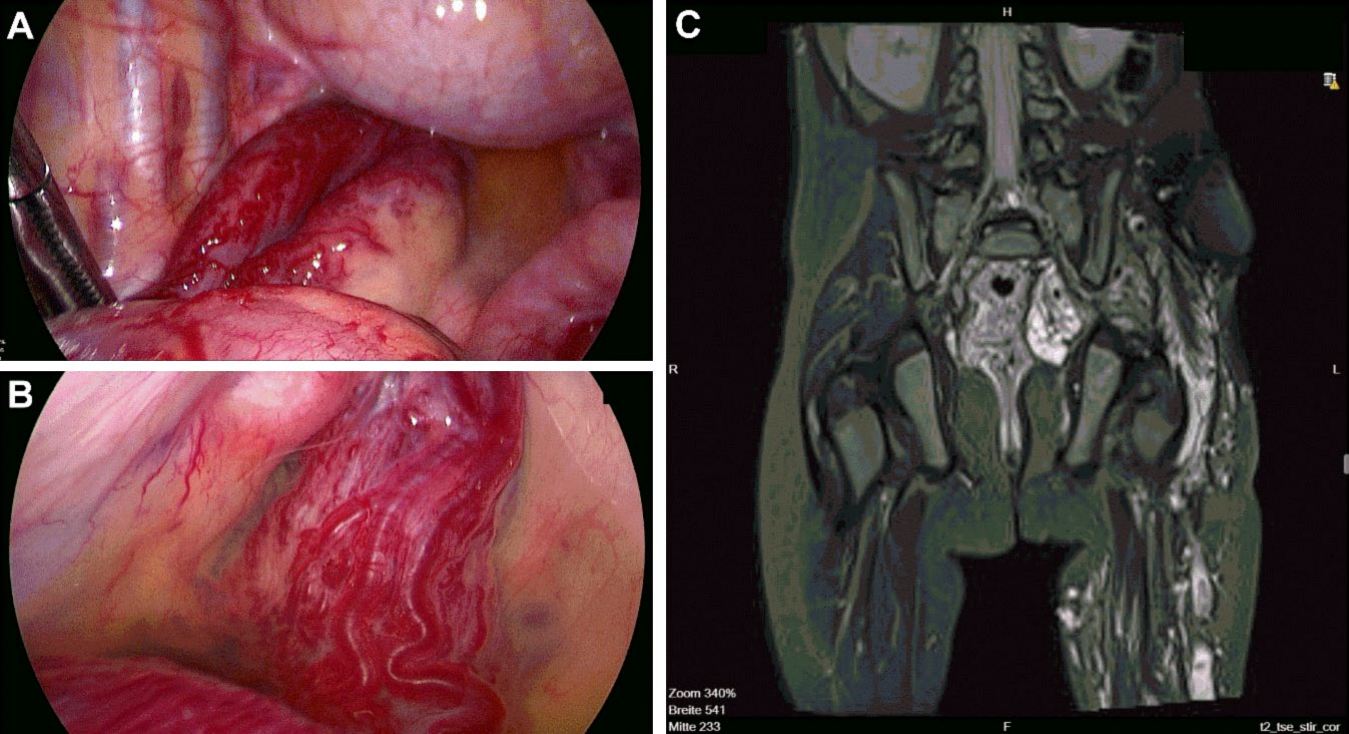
(A) Intraoperative view showing a venous vascular malformation infiltrating the sigmoid colon (visible in the central part of the image). (B) Intraoperative detail of the rectal involvement with diffuse subserosal vascular channels. (C) Coronar T2‐weighted MRI demonstrating the extensive venous malformation extending from the cutaneous capillary malformation on the left thigh through the obturator foramen into the pelvis, involving the rectal and sigmoid wall.
